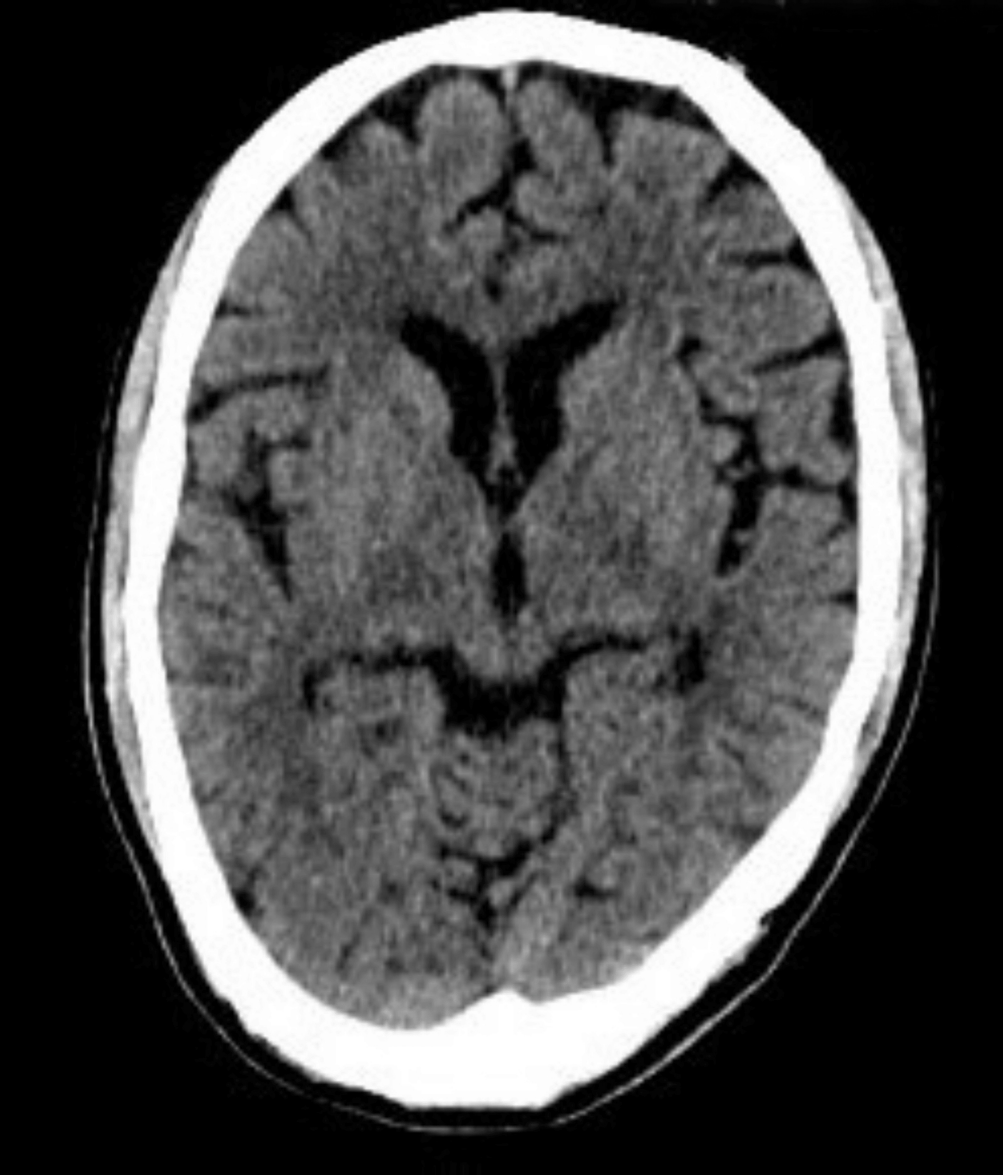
CT scan of the brain (axial section) at the level of basal ganglia (plain study) not showing any abnormality
